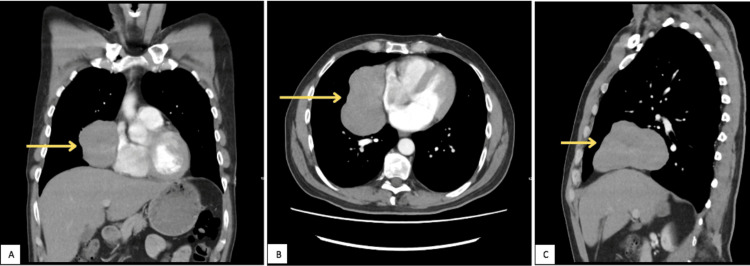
CT chest, soft tissue window; coronal view (A), axial view (B) and sagittal view (C) showing a solid right-sided thoracic mass without intralesional fat, calcification or fibrous tissue (yellow arrow)
